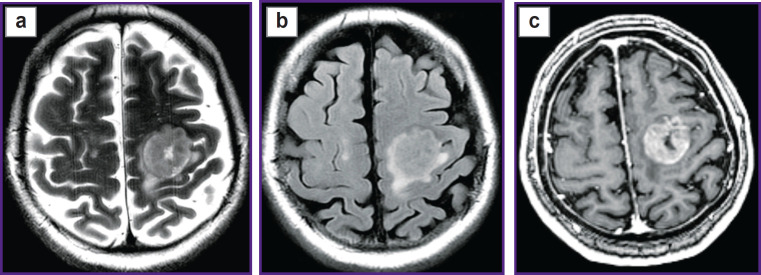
Patient with metastasis of small cell lung cancer in the left posterio-frontal region: (а) Т2-weighted MRI, (b) T2-FLAIR images, (c) contrast-enhanced Т1
